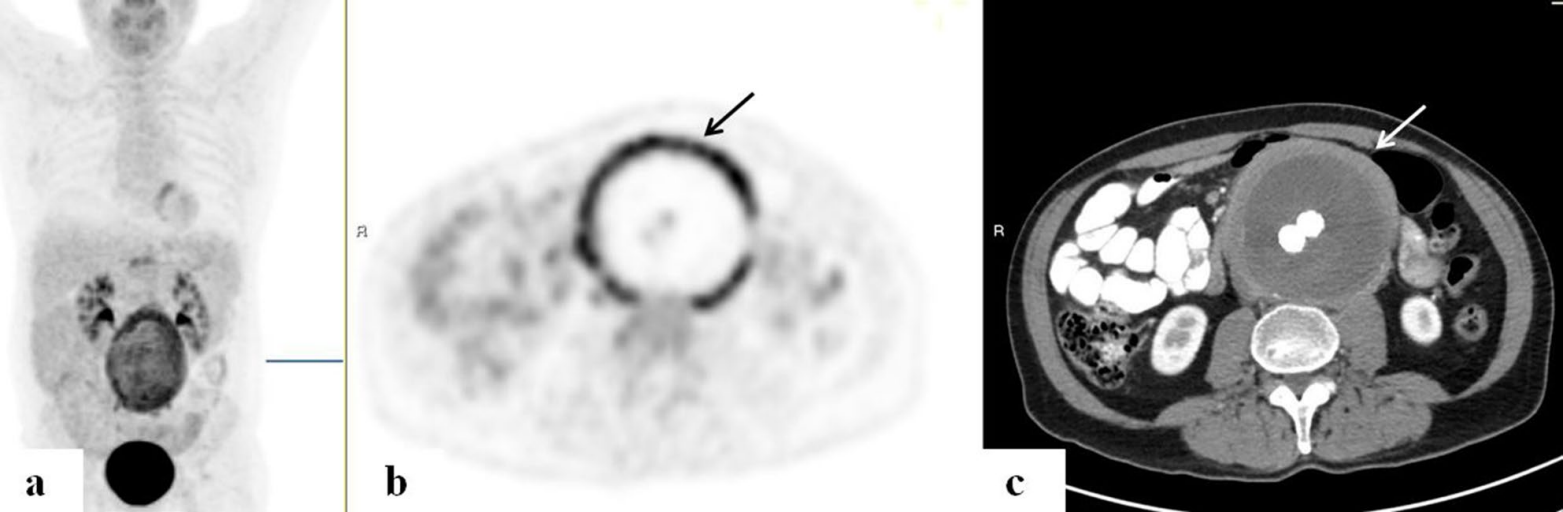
FDG-PET/CT: maximum intensity projection (MIP) (a) a representative PET (b) and CT (c) axial slices. A 65-year-old man with mycotic aneurism of the abdominal aorta. PET demonstrates high intensity of increased uptake (arrows) along the markedly thickened wall of the dilated abdominal aorta following repair.
